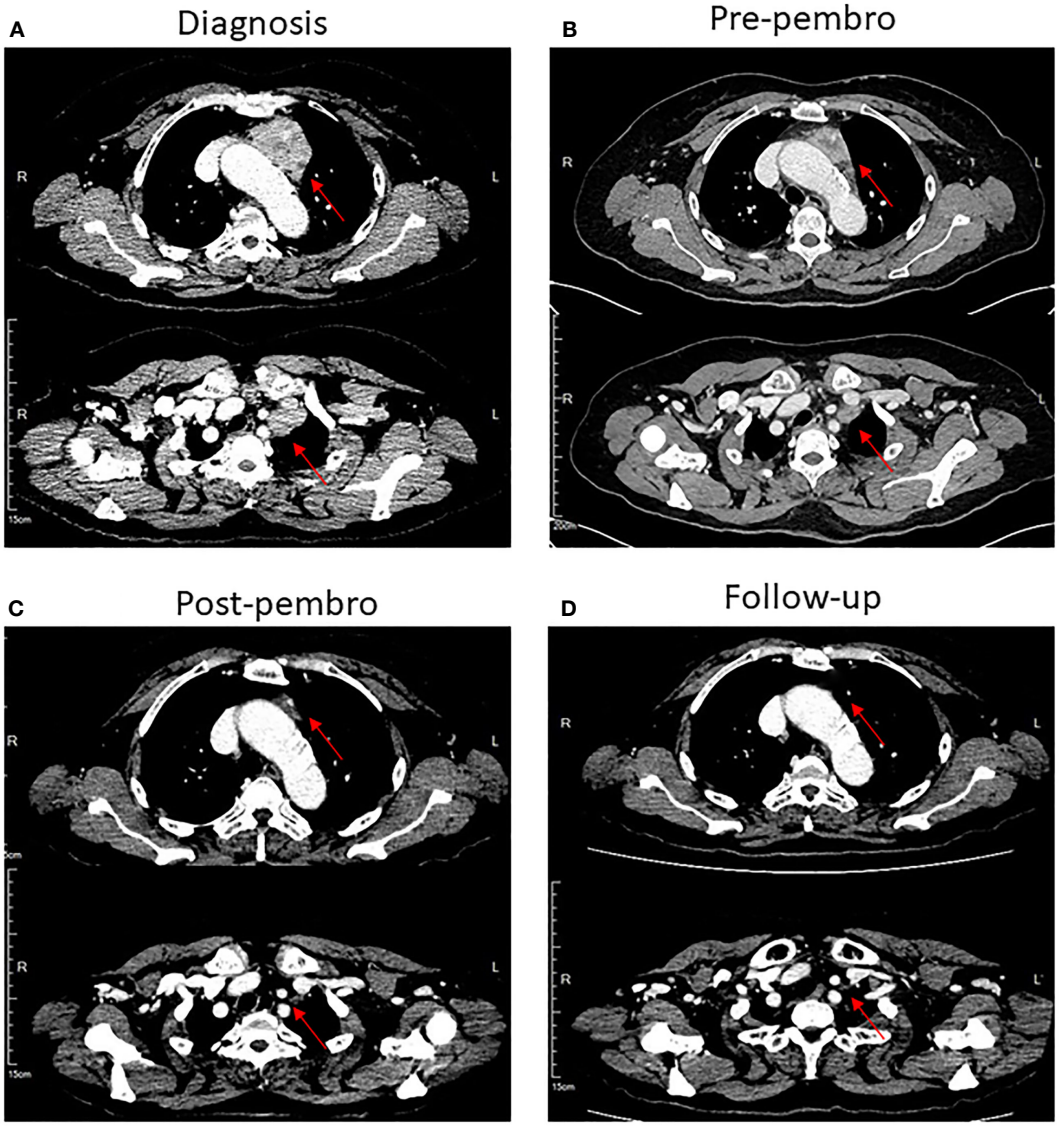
CT scans of the chest showed that patient 1 had a complete radiological response of mediastinal masses after treatment with pembrolizumab in combination with chemotherapy. (A) Mediastinal masses at diagnosis, lesion has been marked with red arrows. (B) Mediastinal masses before treatment with pembrolizumab combined with chemotherapy. (C) Mediastinal masses are markedly improved after treatment with four cycles of pembrolizumab combined with chemotherapy. (D) Disappearance of mediastinal masses in follow-up.
